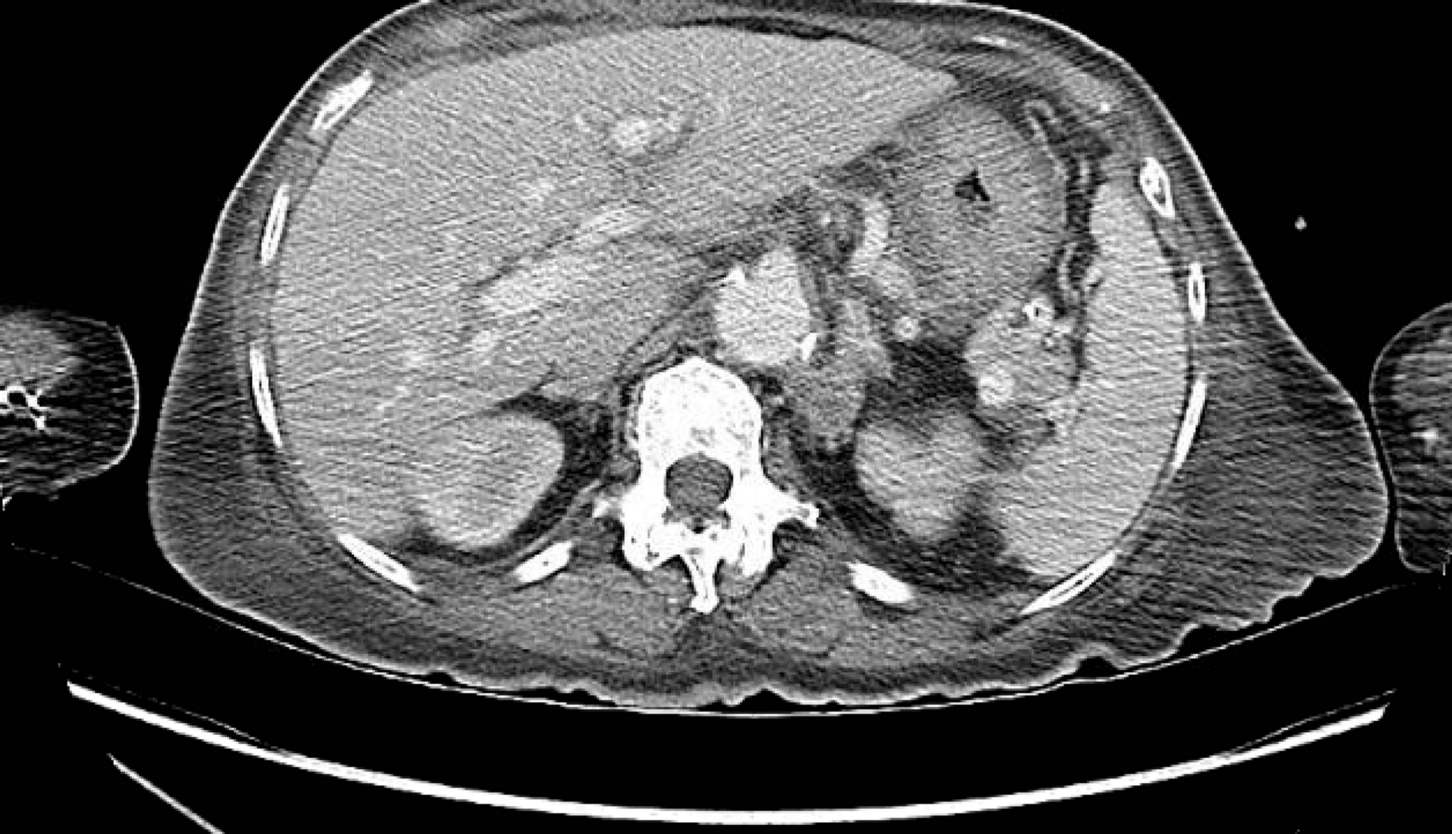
CT abdomen and pelvis. The image shows left retroperitoneal, periaortic lymphadenopathy.
